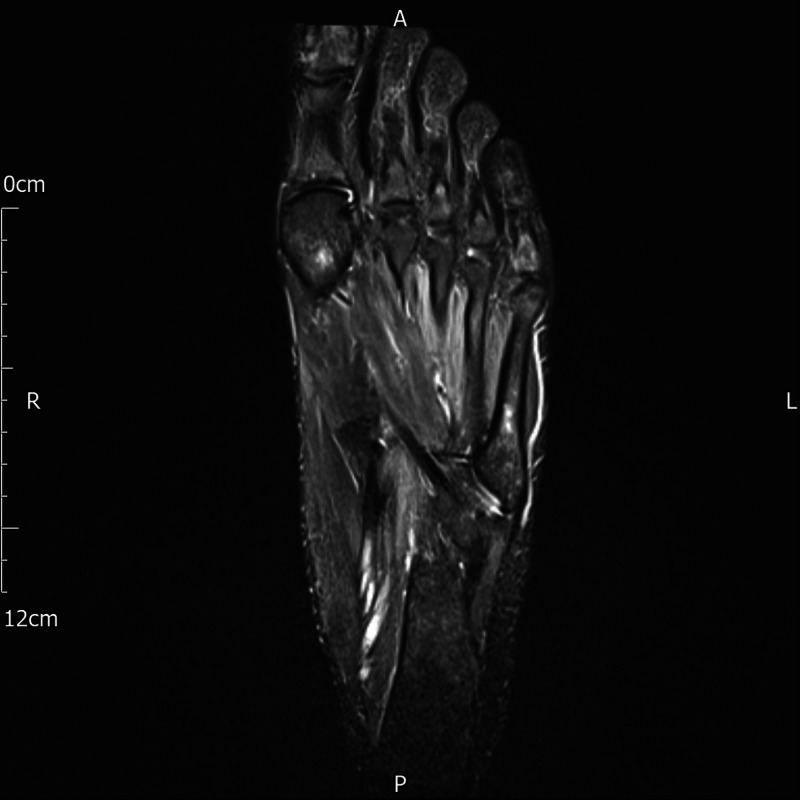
MRI left foot showing stress fractures in first, second, fourth and fifth metatarsals.
